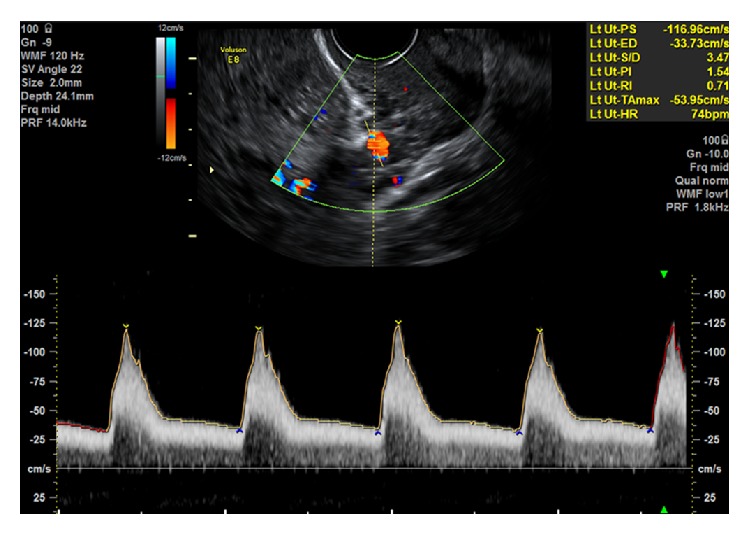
Transvaginal Doppler interrogation of the uterine artery at the cervicocorporeal junction. Normal uterine artery waveforms. Reproduced with permission from Associate Professor F. da Silva Costa.
